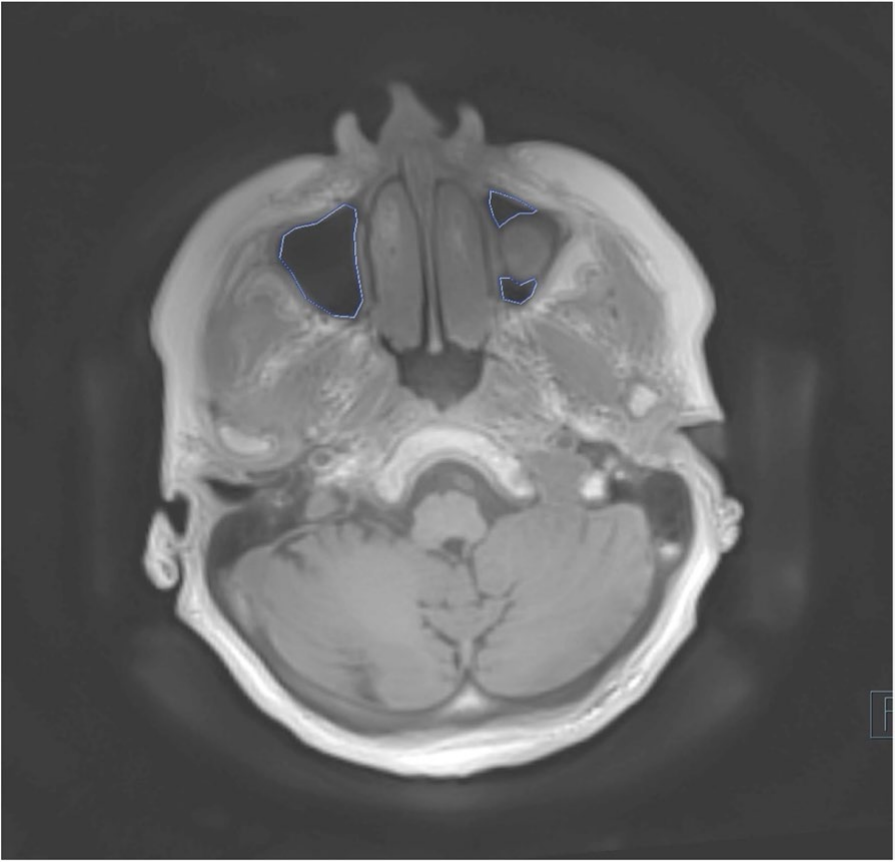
MRI, 6 months postoperatively, marking of the sinus with cut out of the bone graft, transversal layer, T1 weighted petra sequence
